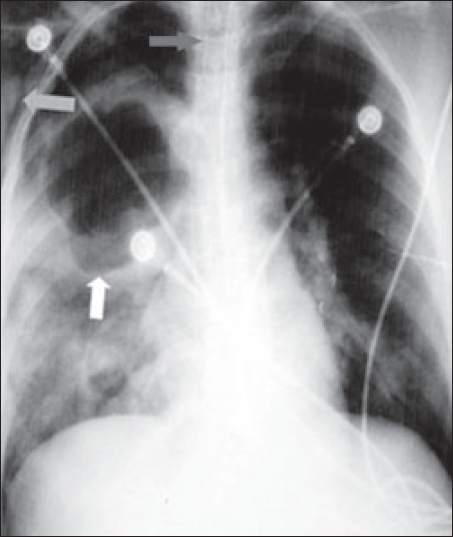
Post intubation chest X-ray showing endotracheal tube (dark gray arrow), ruptured cyst (white arrow), and surgical emphysema (light gray arrow)
